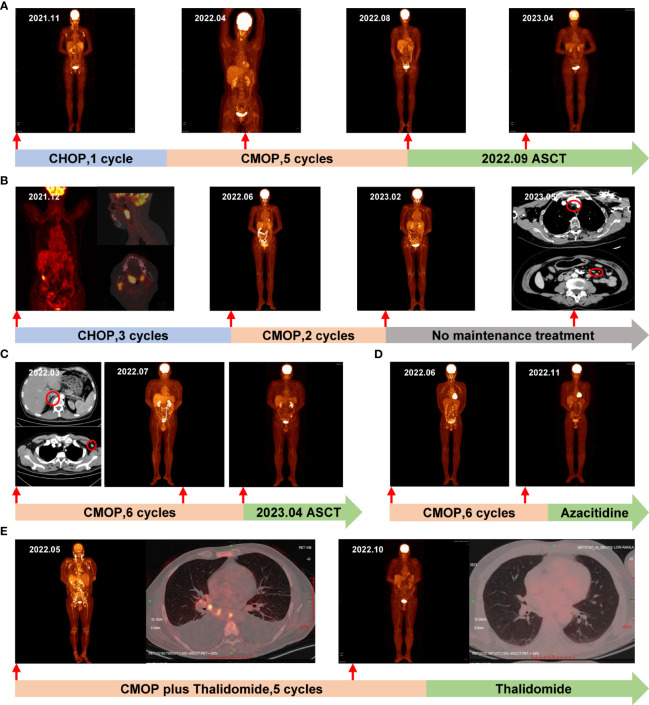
The timeline and representative PET/CT and CT images. Each treatment the patient received is labeled on the graph. (A) Case 1: Before treatment in November 2021, PET/CT revealed increased glucose metabolism in multiple areas, including the neck, chest, abdomen, bilateral inguinal lymph nodes, occiput, right chest, back, and subcutaneous fat nodules on both upper extremities. Following 3 cycles of CMOP, a PET/CT scan in April 2022 indicated CR, which was sustained after 5 cycles of CMOP in August 2022. Notably, in April 2023, PET-CT confirmed a lasting CR post-ASCT; (B) Case 2: In December 2021, the initial PET/CT revealed enlarged tonsils and right cervical lymph nodes with heightened metabolism. Following 3 cycles of CHOP, a PET/CT scan in June 2022 confirmed CR. Subsequently, after 2 cycles of CMOP in February 2023, another PET/CT displayed CR. However, a CT scan in May 2023 showed enlarged lymph nodes in the mediastinum, and abdominal cavity, marked by red circles; (C) Case 3: In March 2022, pre-treatment CT scans revealed enlarged lymph nodes and a nodule on the right side of the thoracic 12 vertebrae, marked by red circles. Subsequently, a PET/CT scan in July 2022 confirmed CR after 4 cycles of CMOP. Another PET/CT in November 2022 validated sustained CR following 6 cycles of CMOP; (D) Case 4: In June 2022, pre-treatment PET/CT revealed multiple lymph nodes in the neck, chest, and abdomen. Subsequent PET/CT in November 2022 demonstrated CR after 4 cycles of CMOP; (E) Case 5: In May 2022, PET/CT revealed increased glucose metabolism in multiple lymph nodes, bones, and an enlarged spleen, along with pleural effusion. By October 2022, after 4 cycles of CMOP plus thalidomide, PET/CT confirmed CR and the pleural effusion had resolved. PET, positron emission tomography; CT, computed tomography; CR, complete remission; ASCT, autologous stem cell transplantation.
